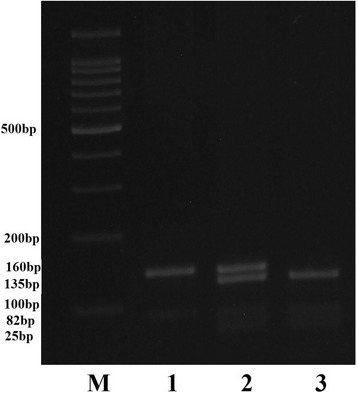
Electrophoresis pattern of PCR-RFLP for detection of *IFNL3* rs12979860 C/T polymorphism. Lane M, DNA marker; lane 1, subjects with homozygote TT genotype; lane 2, subjects with heterozygote CT genotype; lane 3, subjects with homozygote CC genotype
